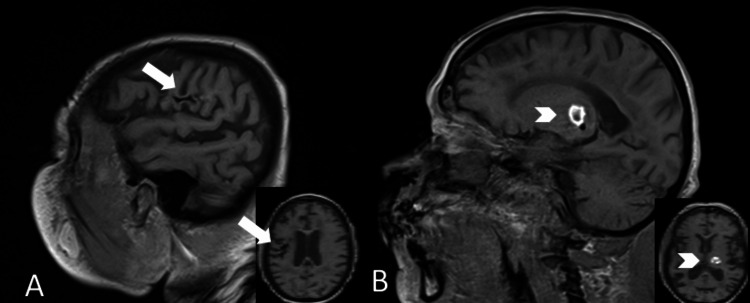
Comparison of T1-weighted MRI signal characteristics of the two lesions on sagittal views with corresponding axial views shown in small windows A: the right temporoparietal lesion (arrow) shows linear subcortical T1 hypointensity consistent with remote hemorrhage, without evidence of new hemorrhage; B: in contrast, the left thalamic lesion (arrowhead) demonstrates focal T1 hyperintensity, consistent with subacute hemorrhage.
